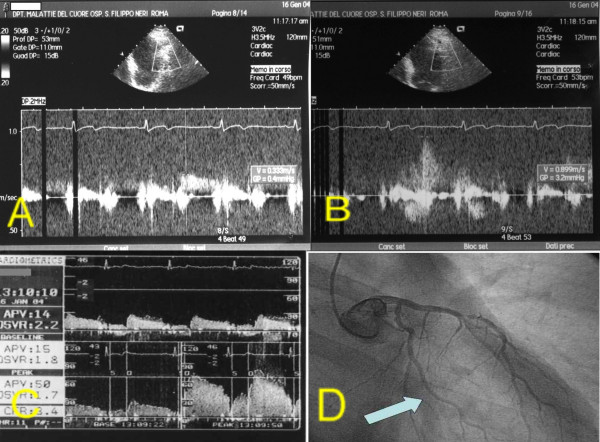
Patients 1. A: TTDE before adenosine. B: TTDE after adenosine. C: DW recording. D: coronary angiography with site of DW recording (arrow). DW: Doppler-wire. TTDE: transthoracic Doppler echocardiography.
